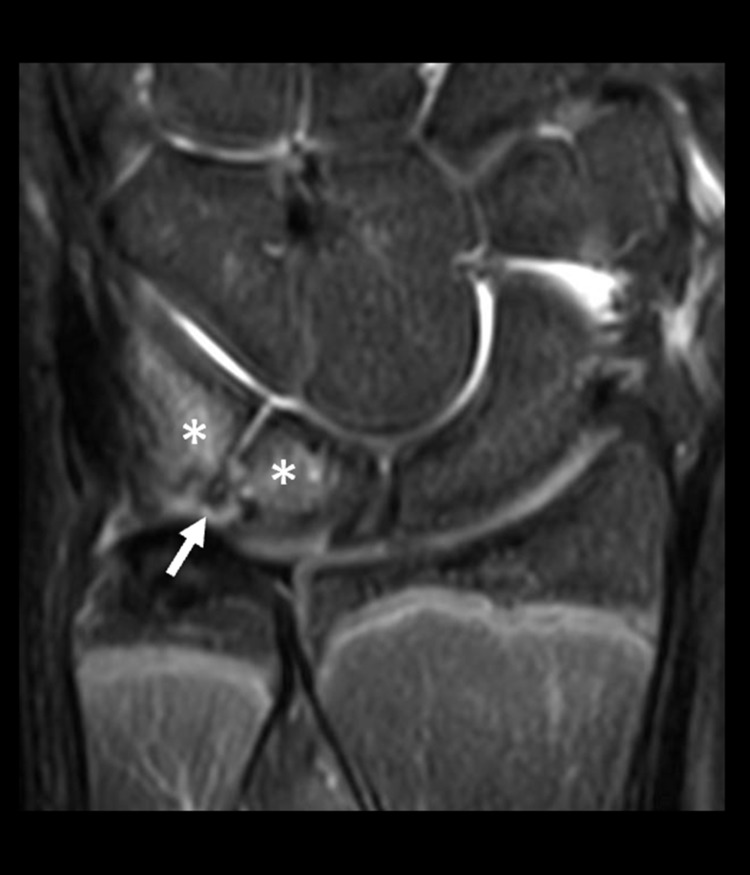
Coronal T2-weighted fat-suppressed magnetic resonance (MR) image from the same MR scan as the prior figure There is edema-like signal (asterisks) within the lunate and triquetrum adjacent to the coalition (solid arrow), consistent with stress changes.
